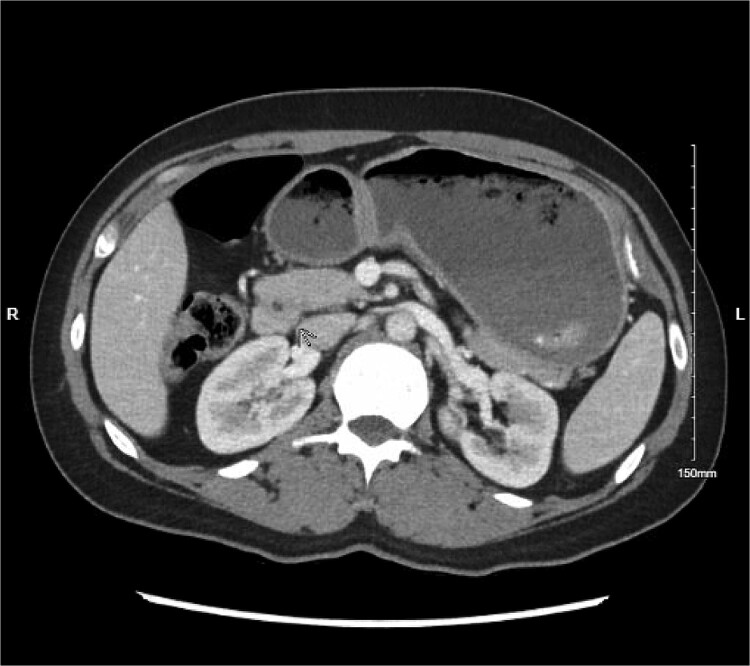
Abdominal CT with IV contrast showing fluid distension of the stomach and duodenal bulb with encirclement of pancreatic tissue around the first portion of the duodenum.
